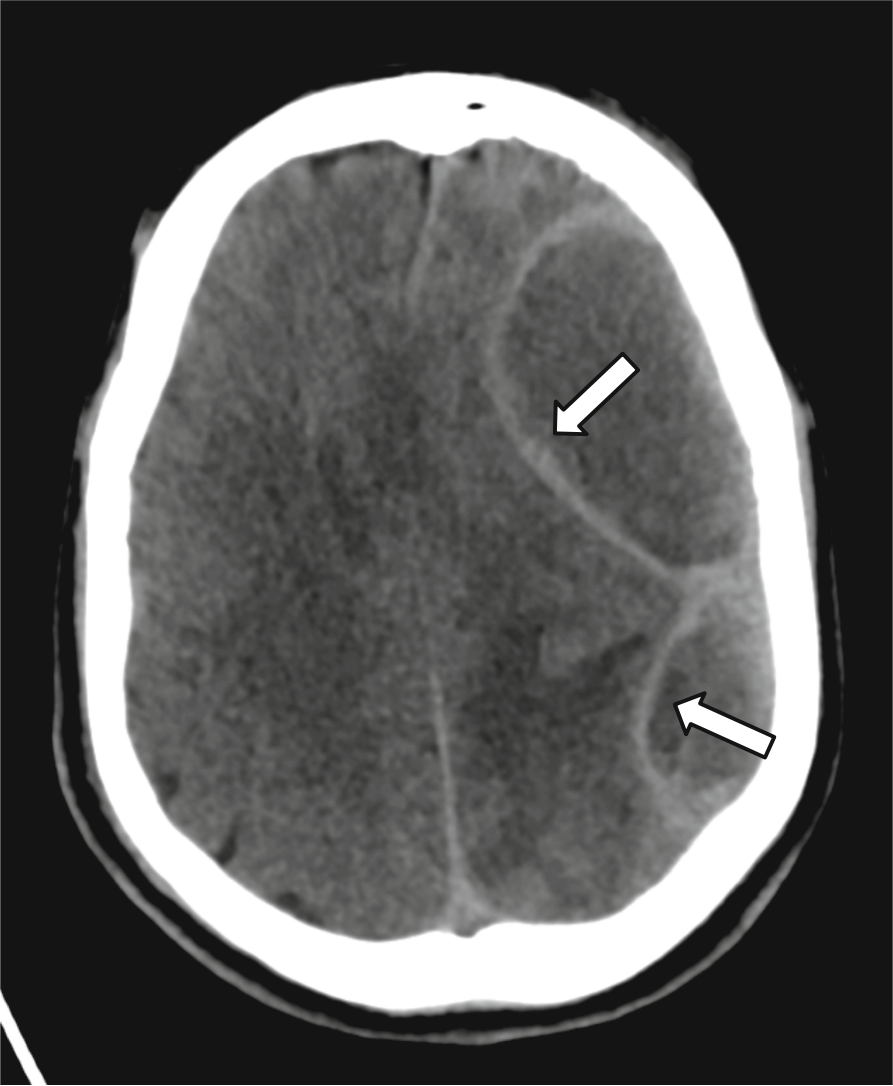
CT brain on admission, axial section. CT brain showed massive left subdural collection (white arrowheads) with mass effect on the adjacent parenchyma
